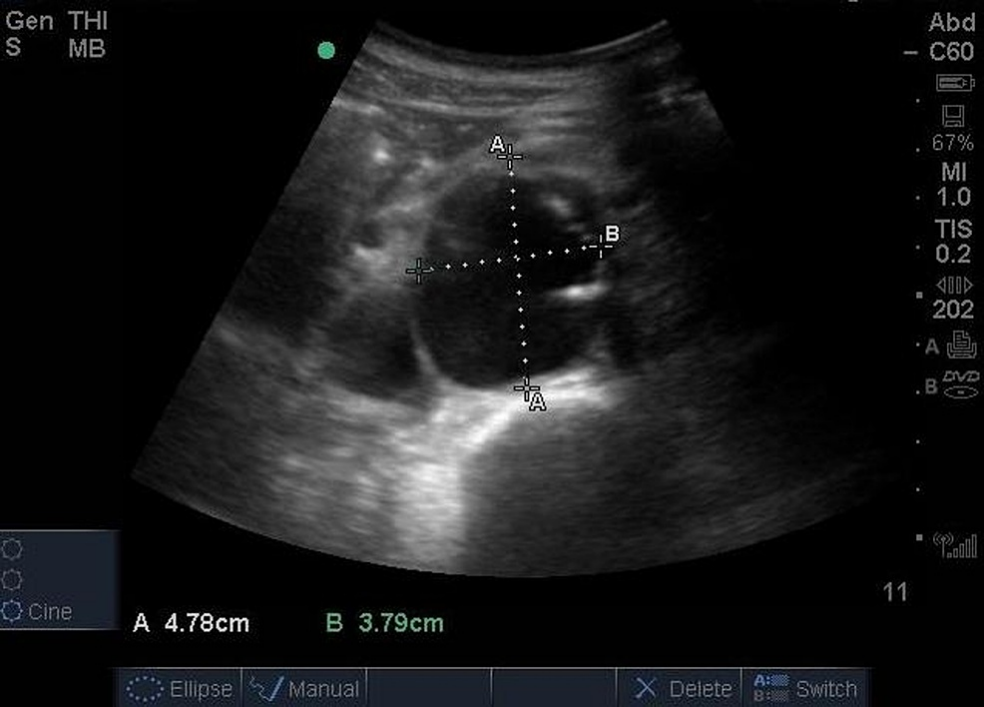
Ultrasound demonstrating abdominal aortic aneurysm measuring 4.78 cm X 3.79 cm on transverse view
